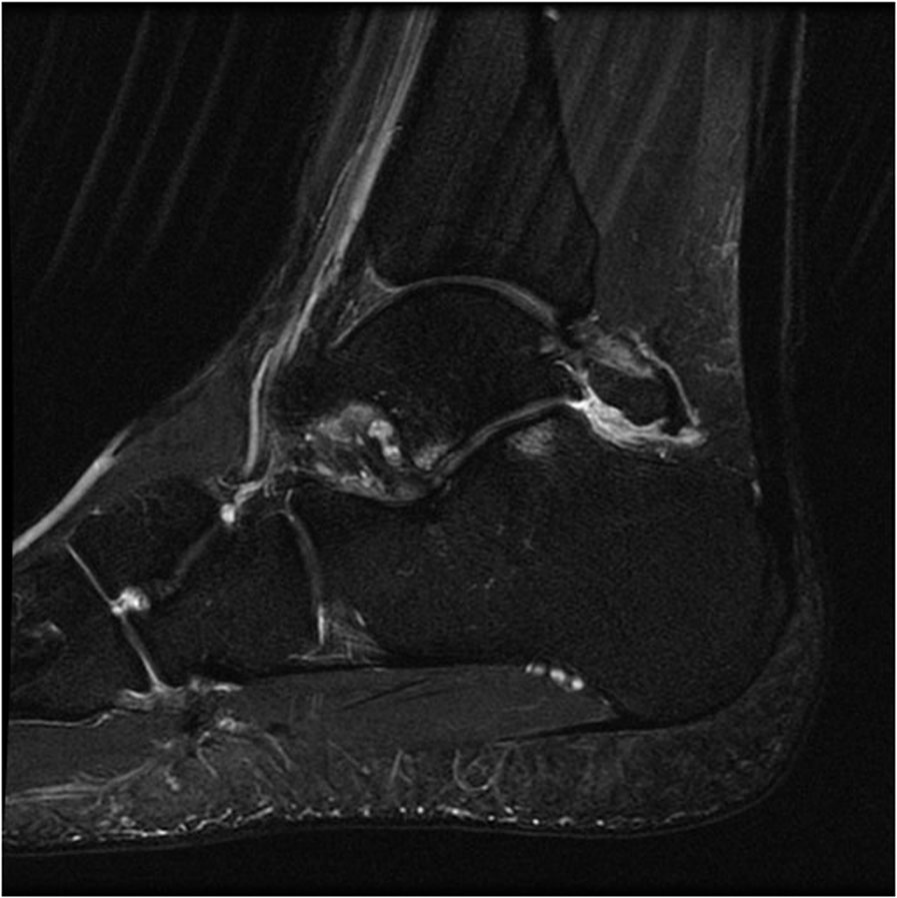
A T2-weighted MRI with reactive edema surrounding an os trigonum
